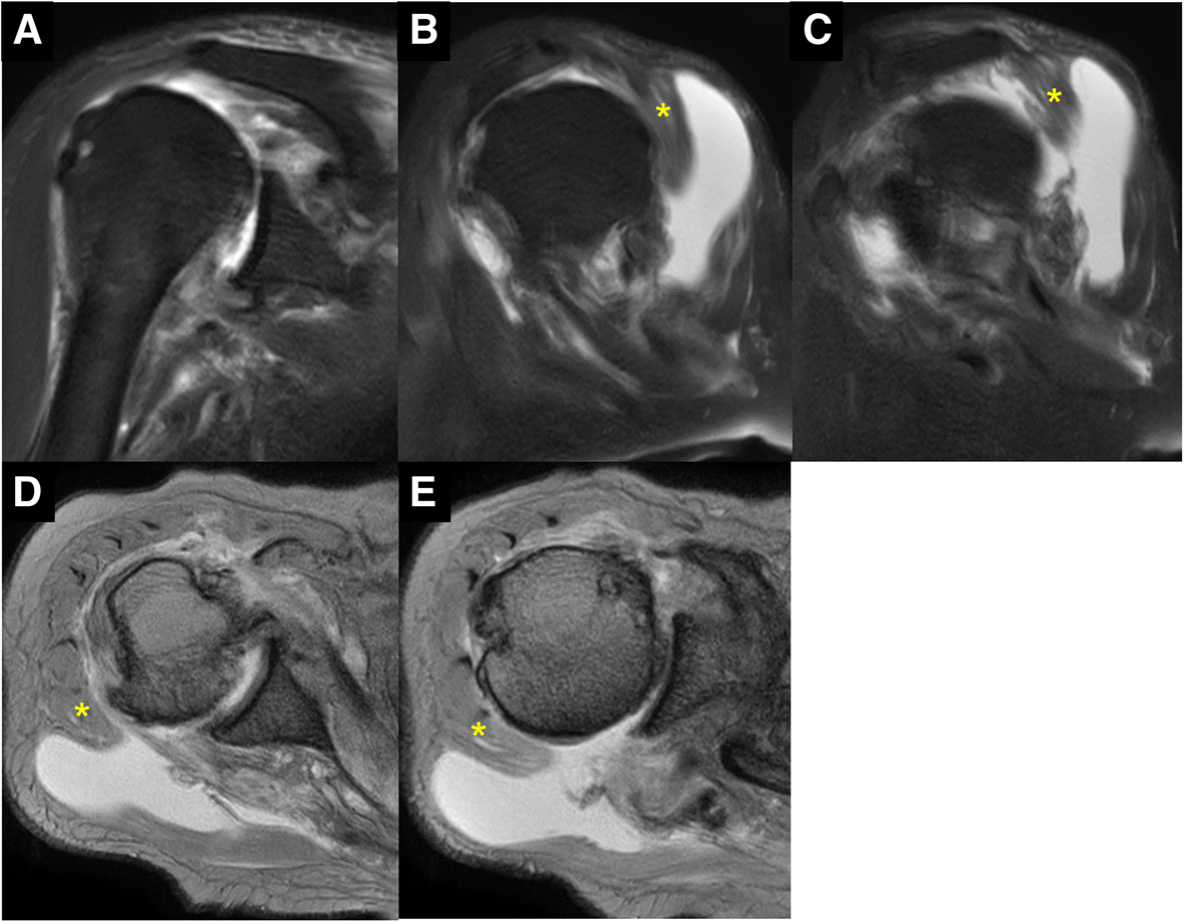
MRI images of the right shoulder 1 week after the closed reduction. Fat-suppressed T2-weighted oblique axial (a) and oblique sagittal (b, c) images, and T2-weighted oblique coronal images (d, e) revealing the complete tear of supraspinatus tendon and infraspinatus tendon, rupture of deltoid posterior fibers (asterisk), and fluid retention in the posterosuperior shoulder. MRI, magnetic resonance imaging
